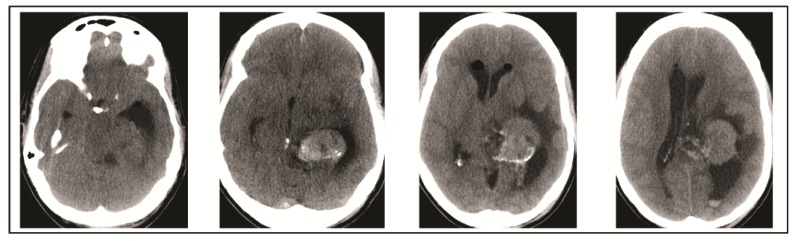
Head CT performed on post-operative day 7 demonstrates increase in cerebral edema, midline shift, and worsened hydrocephalus which prompted operative decompression.
